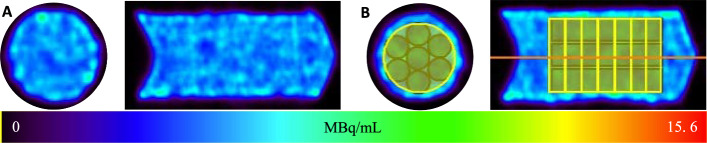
The SPECT images acquired for ^165^Er placed in a syringe with a concentration of 4.6 MBq/mL at the time of acquisition. A) top and side views. B) cylindrical VOIs (dark yellow) drawn for quantitative accuracy and noise calculations and cylindrical VOIs (light yellow) drawn for background calculation. The fly through line used to generate the uniformity profile graph shown on the side view
